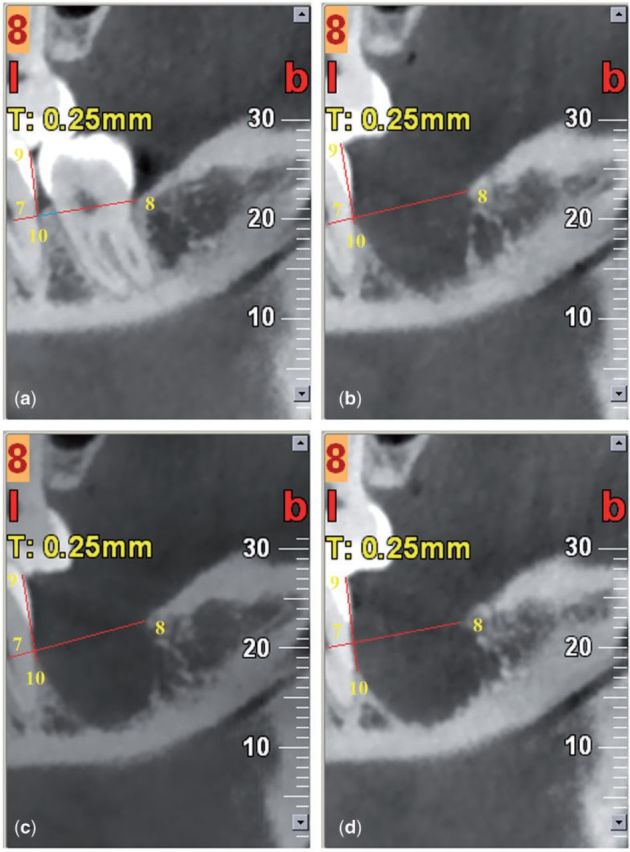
CBCT of experimental site for quantitative analysis of dimensional changes of the alveolar ridge in the mesio-distal direction and in the inter-crestal bone distal to tooth 37. (a): before extraction; (b): 3 months after extraction; (c): 6 months after extraction; (d): 12 months after extraction.
